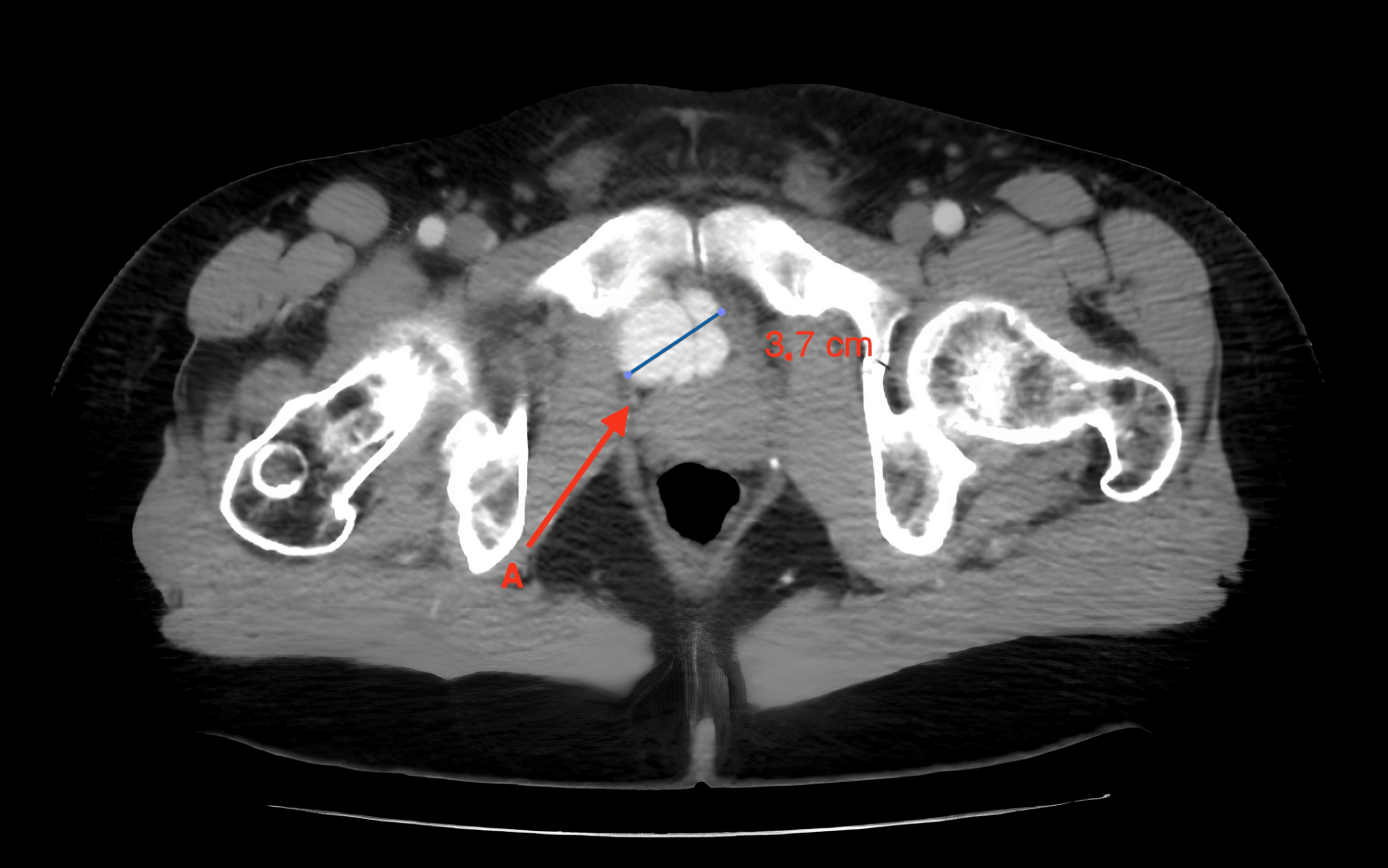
CT angiography. A horizontal section at the level of the symphysis. Up to 3.7 cm aneurysmatic confluent branches of the internal iliac vein can be noted (A).
